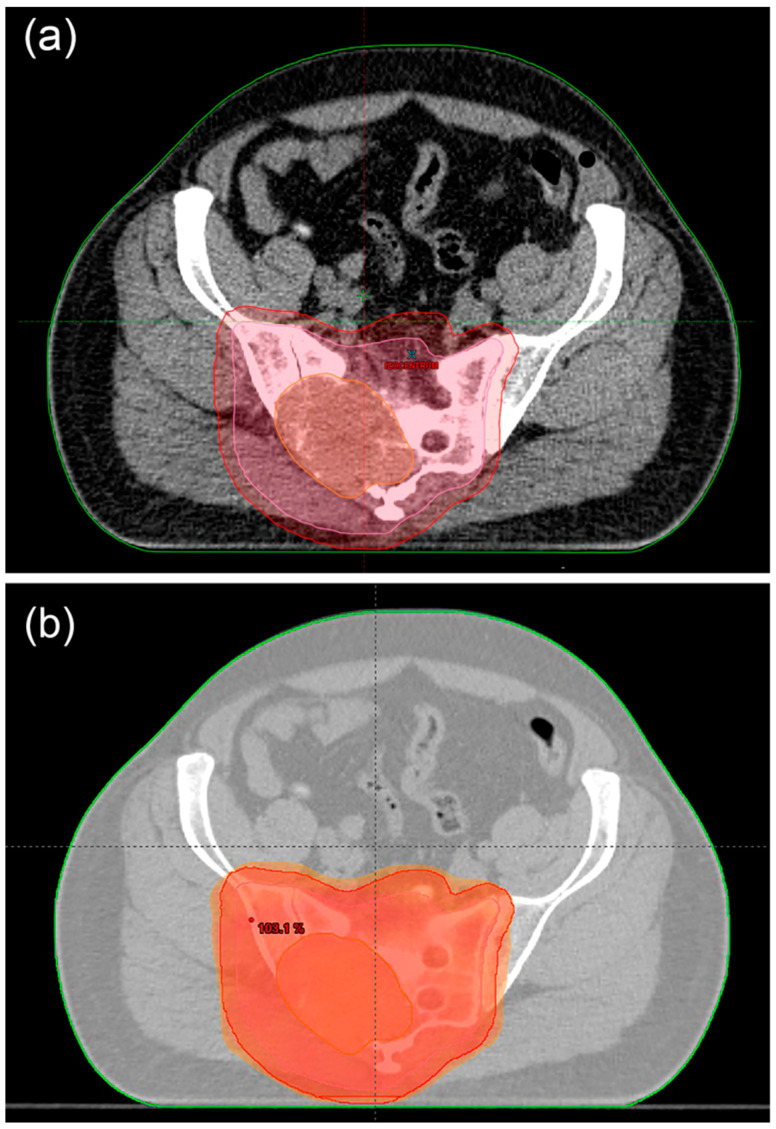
Fifty-year-old patient diagnosed with dedifferentiated chondrosarcoma of the left scapula, at the stage of spread to the sacrum. Received radiation therapy for sacral metastasis. Radiation therapy CT planning (a). Radiation therapy plan (b).
